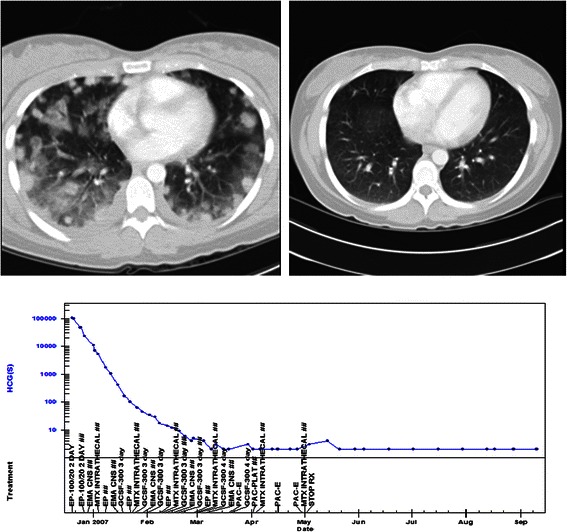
Gestational choriocarcinoma. Pre and post treatment CT scans of the chest showing multiple lung metastases and pulmonary haemorrhage at diagnosis, 12 weeks post partum and a normal scan at completion of chemotherapy. The treatment graph shows the fall and normalisation of the hCG level with combination chemotherapy treatment. The baby was unaffected by the illness, the patient has completed treatment and gone on to have another healthy baby and has been cured of this rare malignancy.
